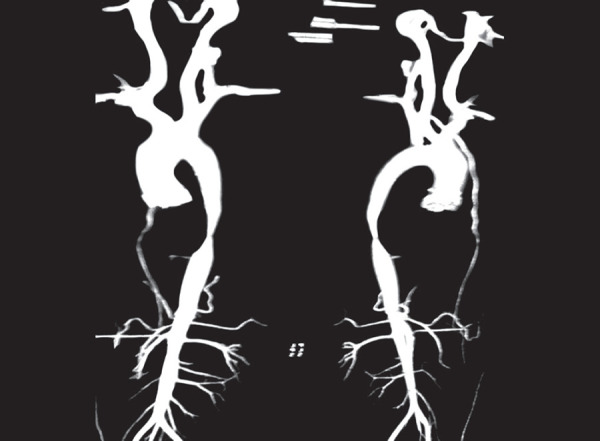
CT angiography of aorta and its branches along with CT abdomen
